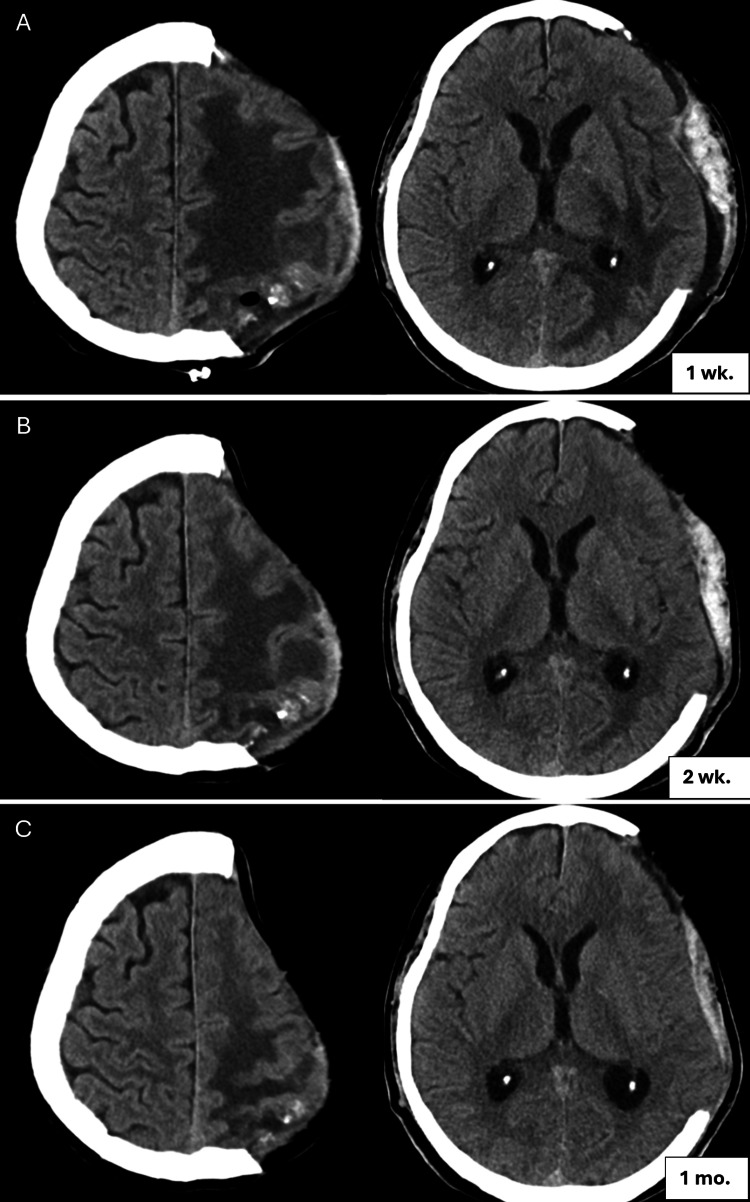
Follow-up cranial CT after surgical removal of CEEH CT: computed tomography; CEEH: chronic encapsulated expanding hematoma Axial views obtained at (A) one week, (B) two weeks, and (C) one month postoperatively demonstrate rapid reduction of vasogenic edema in the left cerebral hemisphere following surgical resection of the CEEH
